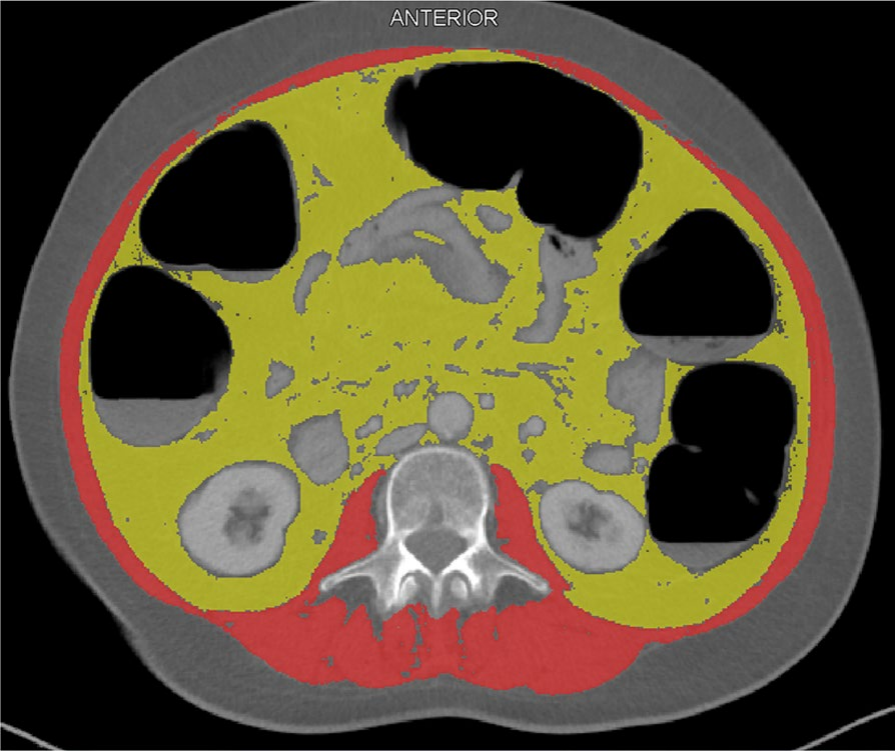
Segmentation of skeletal muscle area (red) and visceral adipose tissue area (yellow) in an axial CT image at the L3 vertebral body using the Slice-O-Matic software
